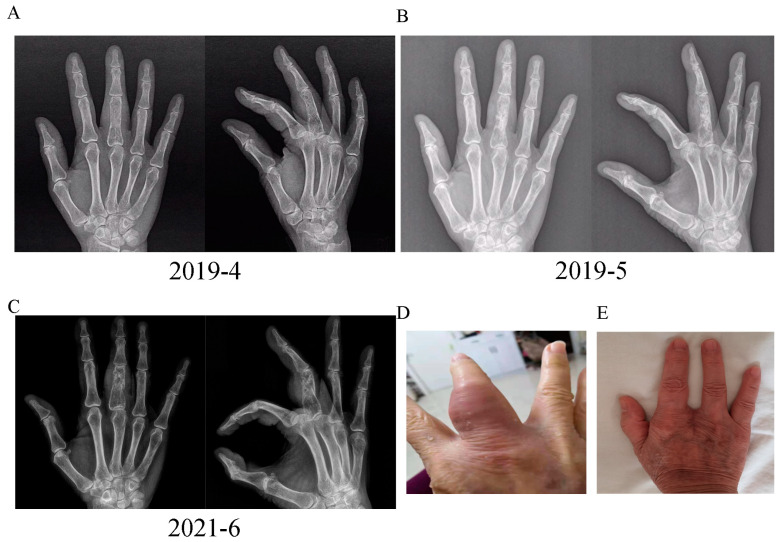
(A) Digital X-ray examination indicated ground glass opacity in the bone marrow cavity and destruction of the bone cortex in the right proximal middle phalanx in 3 April 2019. (B) Digital X-ray examination was performed in 22 May 2019; this indicated high density opacity which was the bone graft in the right proximal middle phalanx. (C) Digital X-ray examination indicated hyperplastic and pathological fracture-like manifestations in the right proximal middle phalanx. (D) The swollen middle phalanx of the right hand prior to amputation surgery. (E) The right hand after amputation surgery.
